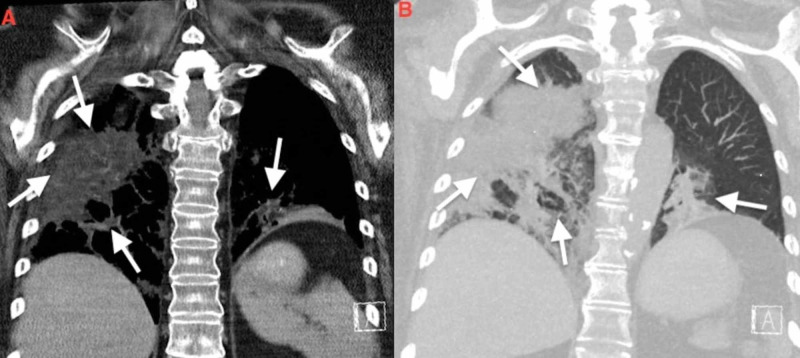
CT Chest showing multifocal, bilateral, consolidative opacities with areas of low density ranging from -91 HU to -47 HU involving bilateral lower lobe and right upper lobe (white arrows in panel A and B) CT: Computed tomography
